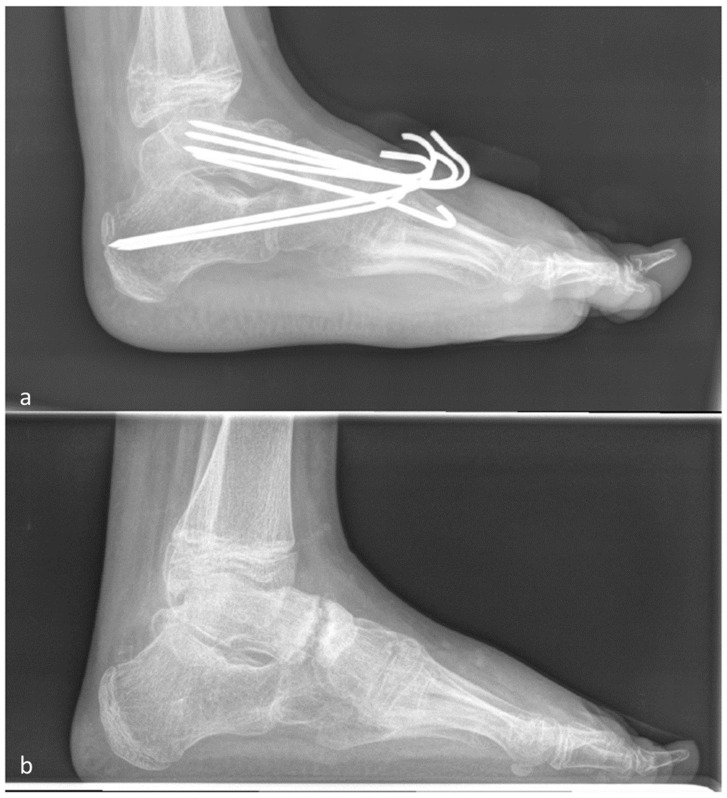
(a) First post-surgery X-ray after talo-navicular and CC arthrodesis with K-wires. (b) Talo-navicular non-union 8 months after surgery.
